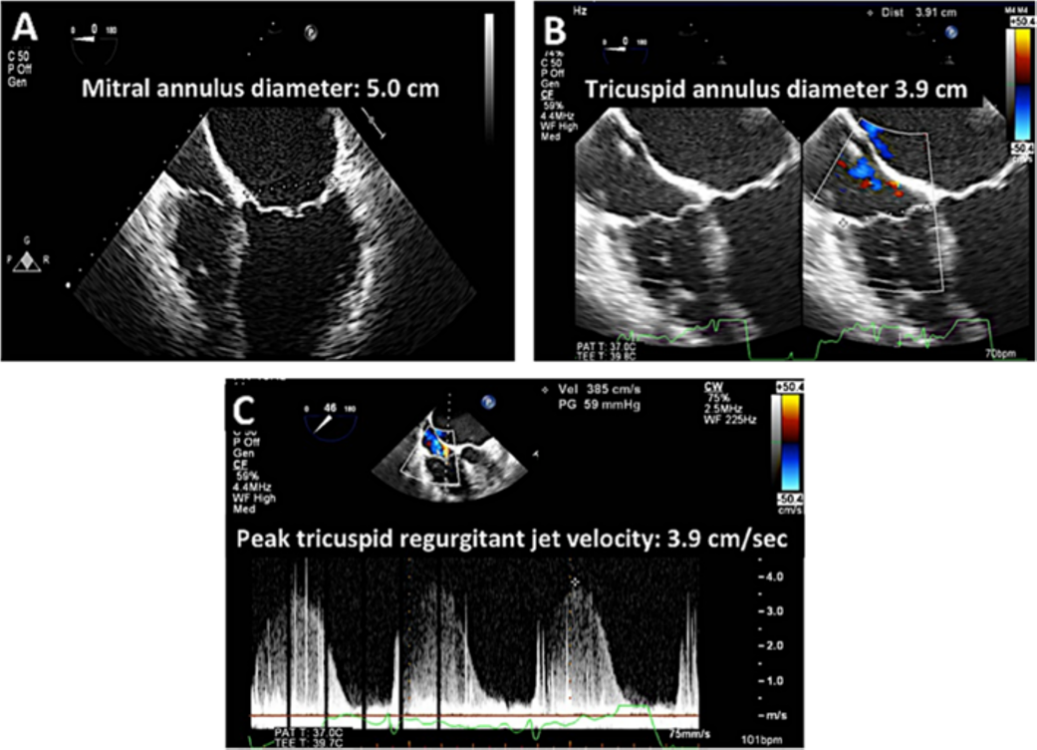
2D assessments during preoperative TEE for MR. Panel A: The mitral valve annulus is dilated (5.0 cm). Panel B: Tricuspid annulus should be measured as this may predict postoperative functional tricuspid regurgitation. In addition, the severity of tricuspid regurgitation should be assessed. Panel C: Maximum TR velocity should be measured to determine pulmonary artery systolic pressure. This is sometimes challenging on TEE and maybe better appreciated on transthoracic imaging.
